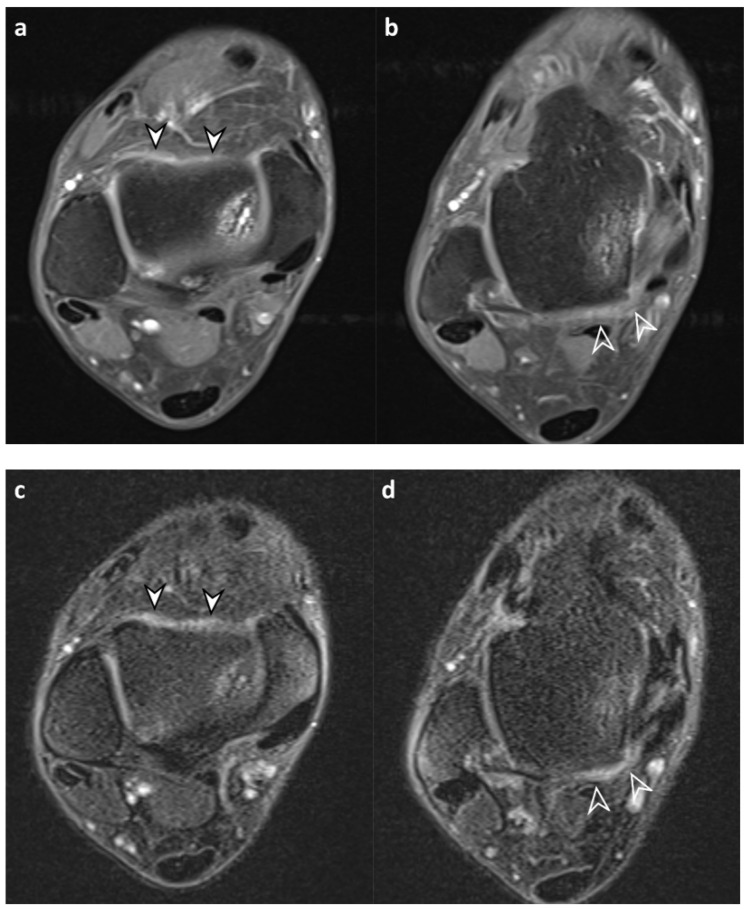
A 60-year-old male with osteochondral lesion of the talus. (a,b) Axial contrast-enhanced, T1-weighted sequence (CE-T1) with fat suppression images reveal enhanced synovium in the anterior recess (solid arrowheads) and posterior recess (open arrowheads). Both readers assigned a synovial visibility grade of 4 (fully visible synovium with good tissue contrast) in all joint compartments. Both readers assigned a synovial thickness score of 0 (maximum thickness < 2 mm) in all joint compartments. (c,d) Corresponding axial fluid-attenuated inversion recovery sequence with fat suppression (FLAIR-FS) images in the same level demonstrate hyperintense synovium at the location corresponding with CE-T1 images. Note that synovium appears thicker with better tissue contrast compared to CE-T1 images. Both readers assigned a synovial visibility grade of 4 (fully visible synovium with good tissue contrast) in all joint compartments. For synovial thickness score, reader 1 assigned 0, 0, 0, and 1 (maximum thickness 2–4 mm) and reader 2 assigned 1, 0, 0, and 1 in the anterior recess, anteromedial gutter, anterolateral gutter, and posterior recess, respectively.
